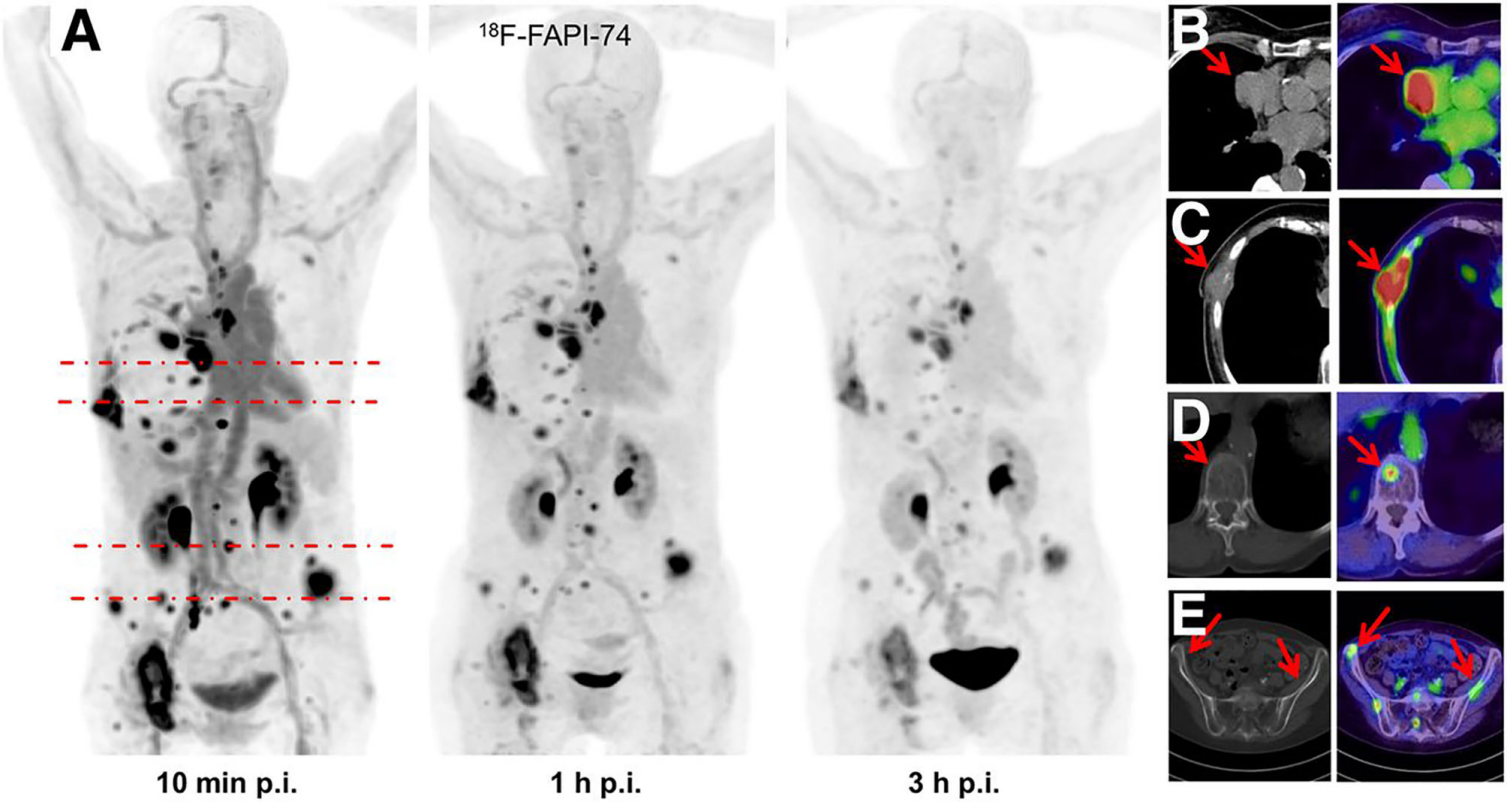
One patient with non–small cell lung cancer who developed systemic metastasis. (A) Maximum intensity projections at 10 min, 1 h, and 3 h after injection of ^18^F-FAPI-74 PET. (B) The uptake of ^18^F-FAPI-74 was significantly different between tumor and normal myocardium. (C) FAPI-positive lesions were confirmed on CT examination. (D, E) Some bone metastases were detected only by FAPI PET. All highlighted arrows represent FAPI uptake in relation to morphology (20).
